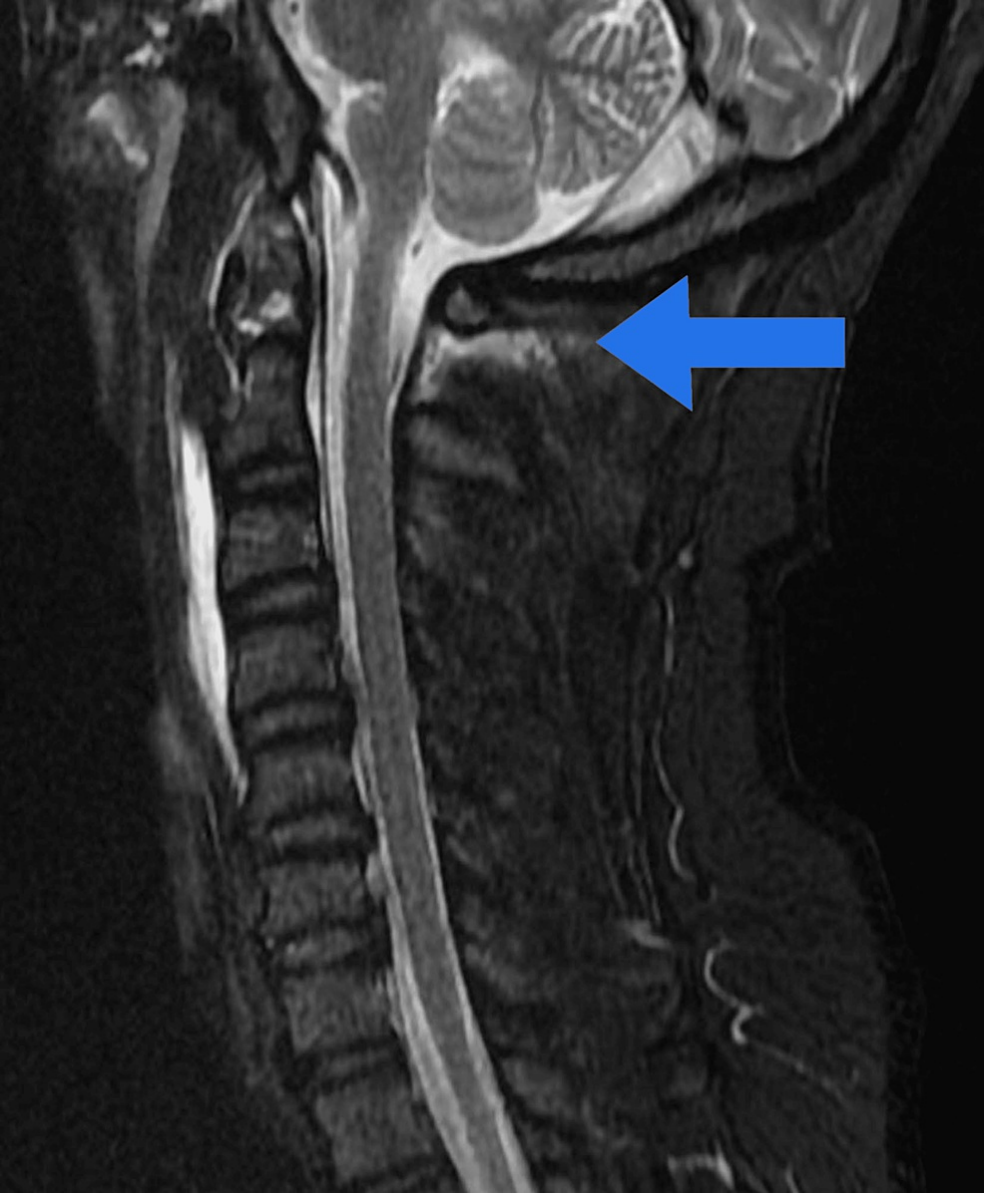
A cervical MRI showing ligamentous injury of the C1 fracture. MRI, magnetic resonance imaging
